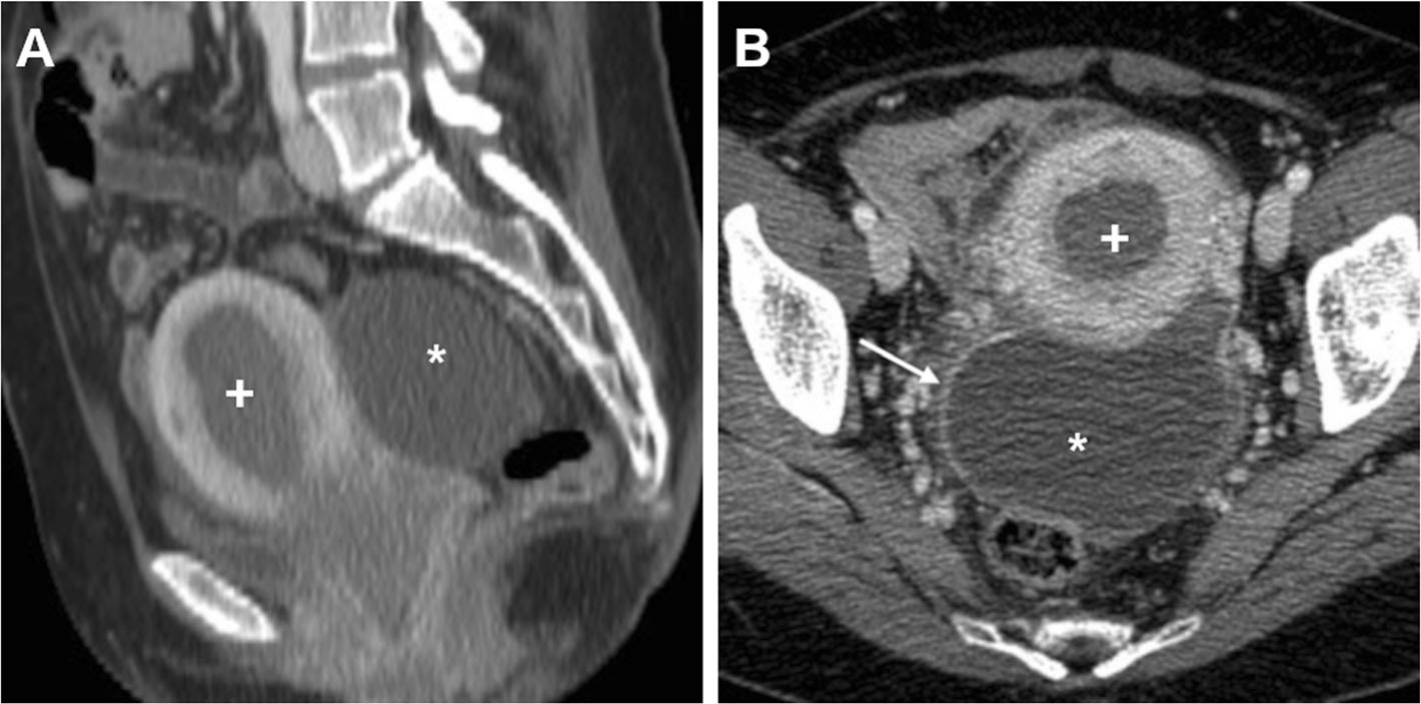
Haematometra and endometritis at CT. Sagittal (a) and axial (b) postcontrast CT images show markedly dilated uterine cavity (plus sign) filled by heterogeneous fluid. Associated loculated effusion (asterisk) in the pelvic cul-de-sac with thin serosal hyperenhancement (thin arrow in b)
